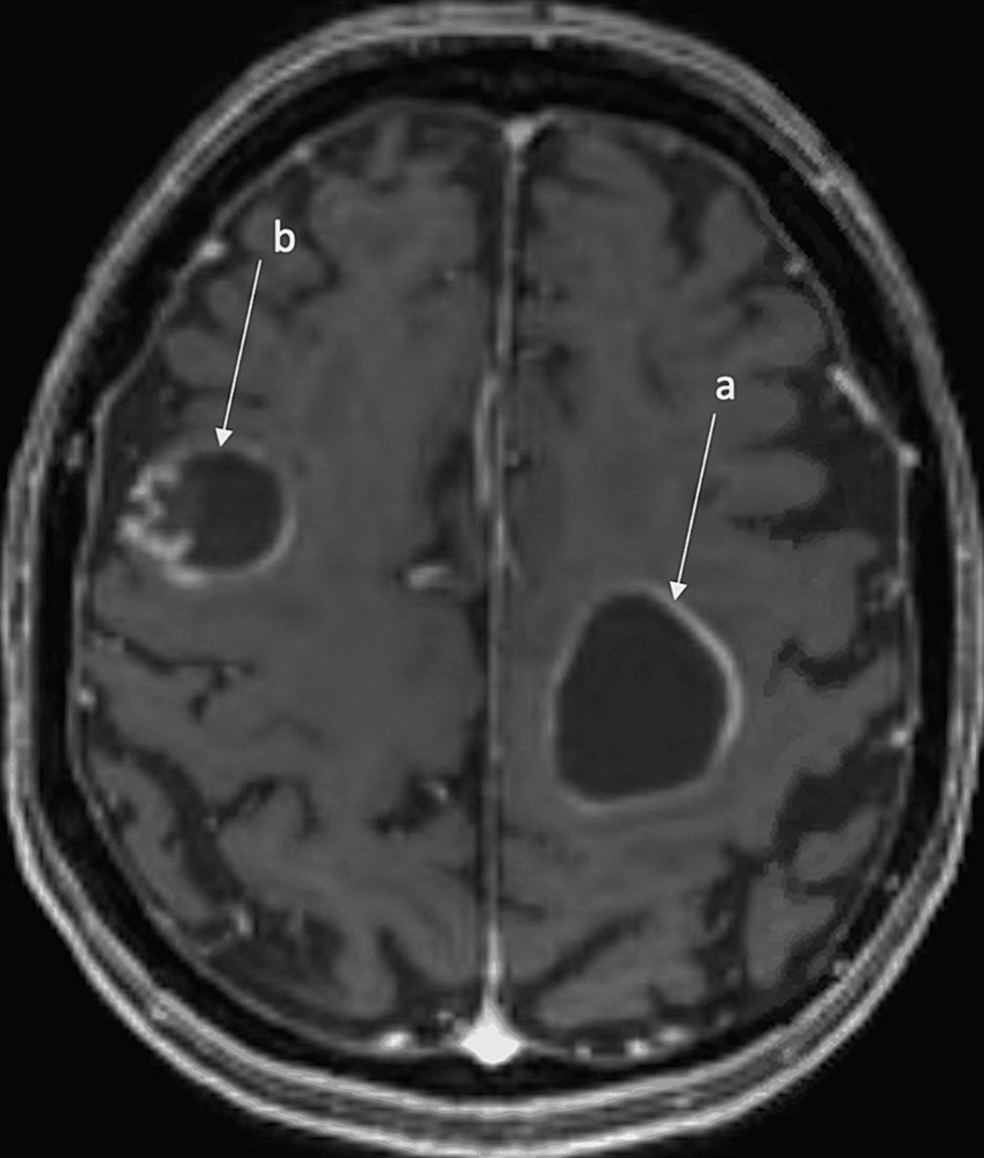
Pre-operative T1-weighted axial magnetic resonance imaging (MRI) view of the brain with contrast, preoperative scan shows (a) 31 x 30 mm cystic lesion in the frontal lobe with rim enhancement inferiorly and (b) 18 x 18 mm cystic lesion in the high frontal region with peripheral enhancement ring along the lateral border.
